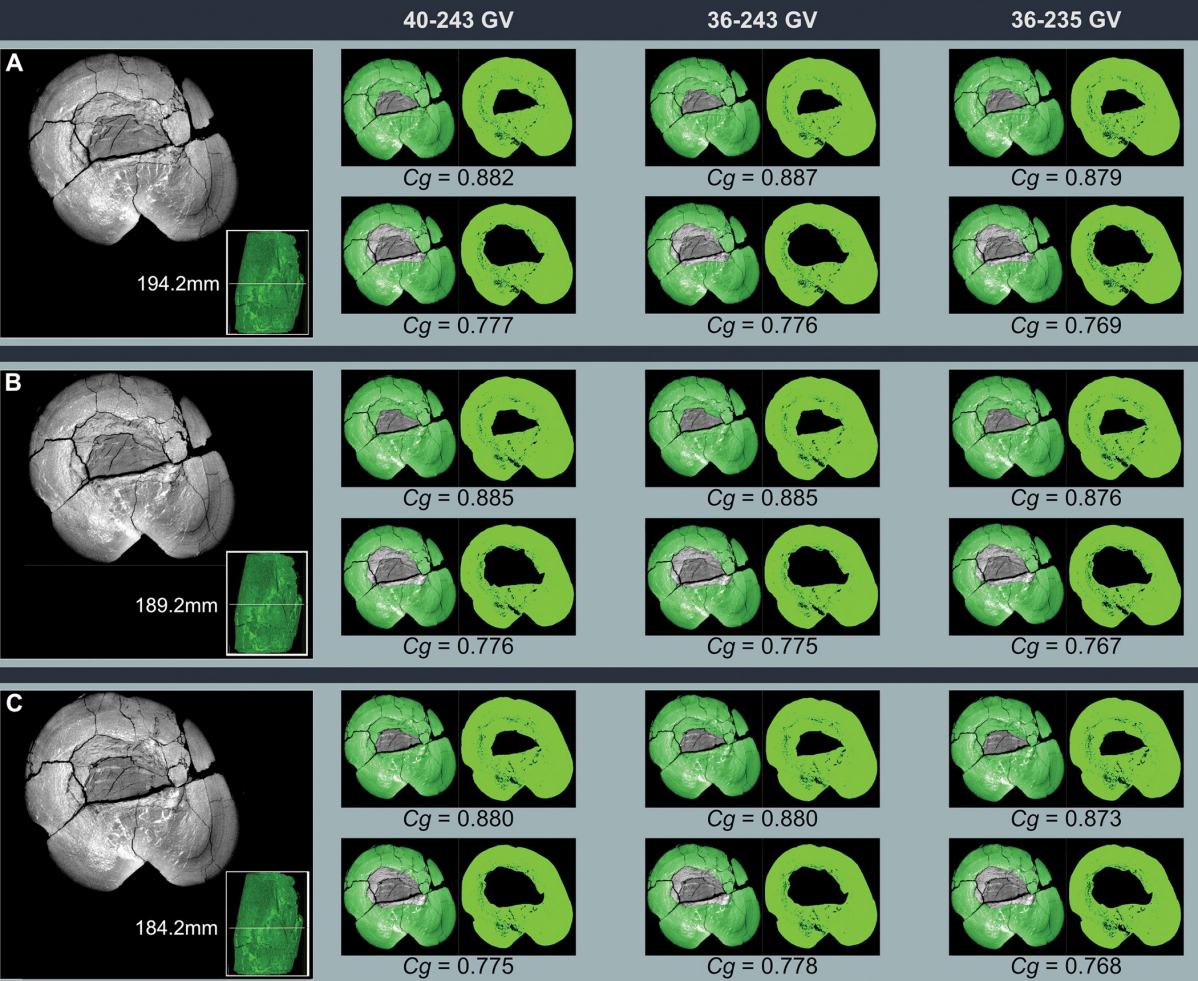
Cg* measured in three adjacent CT cross sections through the distal shaft of the right femur of the spinosaurid *Baryonyx walkeri* (NHMUK 9951). CT sections (A–C, posterior aspect of femur oriented toward bottom) were taken in successively in more distal positions across 1 cm of the distal shaft of the femur in the portion of the shaft used by Fabbri *et al*. [15: S3E Fig] for their best estimate of *Cg*. The small inset view shows distal end of the femur in medial view with mm distance from the bottom of the radiograph provided by Fabbri *et al*. To the right are three gray-value (GV) thresholds (left to right: 40–243, 36–243, 36–235) capturing a reasonable range of values that might be selected by researchers to binarize the radiograph. For each threshold, masking of the matrix infilling of the medullary space is shown in transparent (left) and binarized (right) views. Option 1 (top row) attempts to replicate medullary masking as published by Fabbri *et al*. Option 2 (bottom row) eliminates additional medullary material that we confirmed from the CT scan as matrix infill rather than cancellous medullary bone. Fabbri *et al*. reported a *Cg* of 0.876. The *Cg* range for our three slices in the vicinity of their preferred CT section using their masking is 0.873–0.887 (mean 0.880); their *Cg* measure is near the low end of that range. The *Cg* range with our masking is 0.767–0.778 (mean 0.773), considerably lower than their *Cg* measure.
